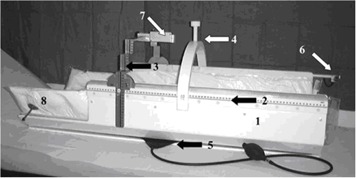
The Elekta stereotactic body frame (SBF). (1) Sidewall containing oblique and horizontal copper wires for CT‐based measurement of longitudinal stereotactic coordinate, the same system is available with copper sulfate fill for use in MRI. (2) Longitudinal stereotactic scale. (3) Stereotactic arc for lateral and AP coordinates. (4) Arc and scaled screw for diaphragm control. (5) Level control. (6,7) SBF attached laser system (leg and trunk) for assistance at patient repositioning. (8) Vacuum pillow. (Taken from Wulf^(9)^)
